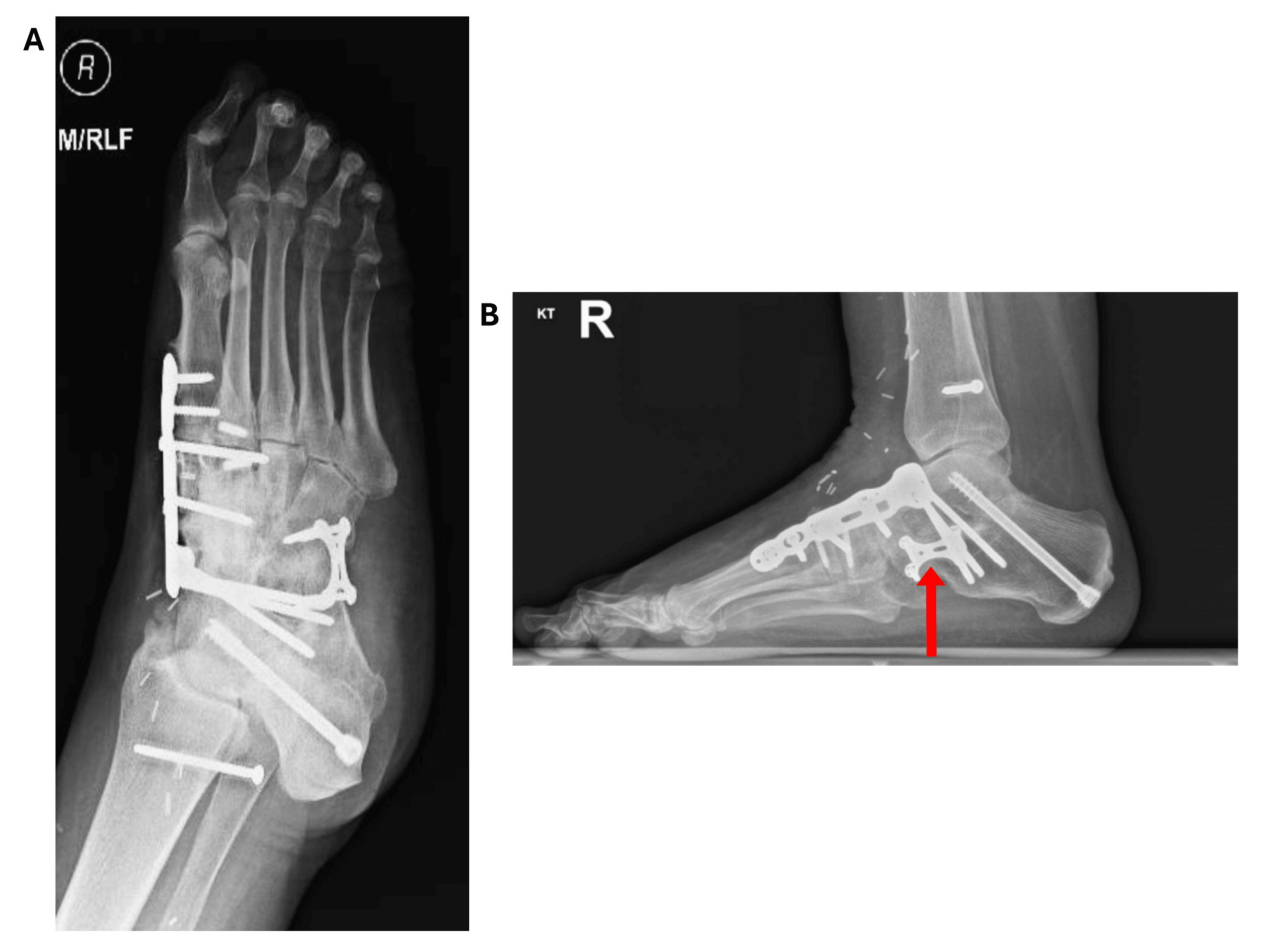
A) Oblique and B) lateral radiographs taken at two-year follow-up demonstrating successful incorporation of the bone graft and intact metalware, marked by an arrow
